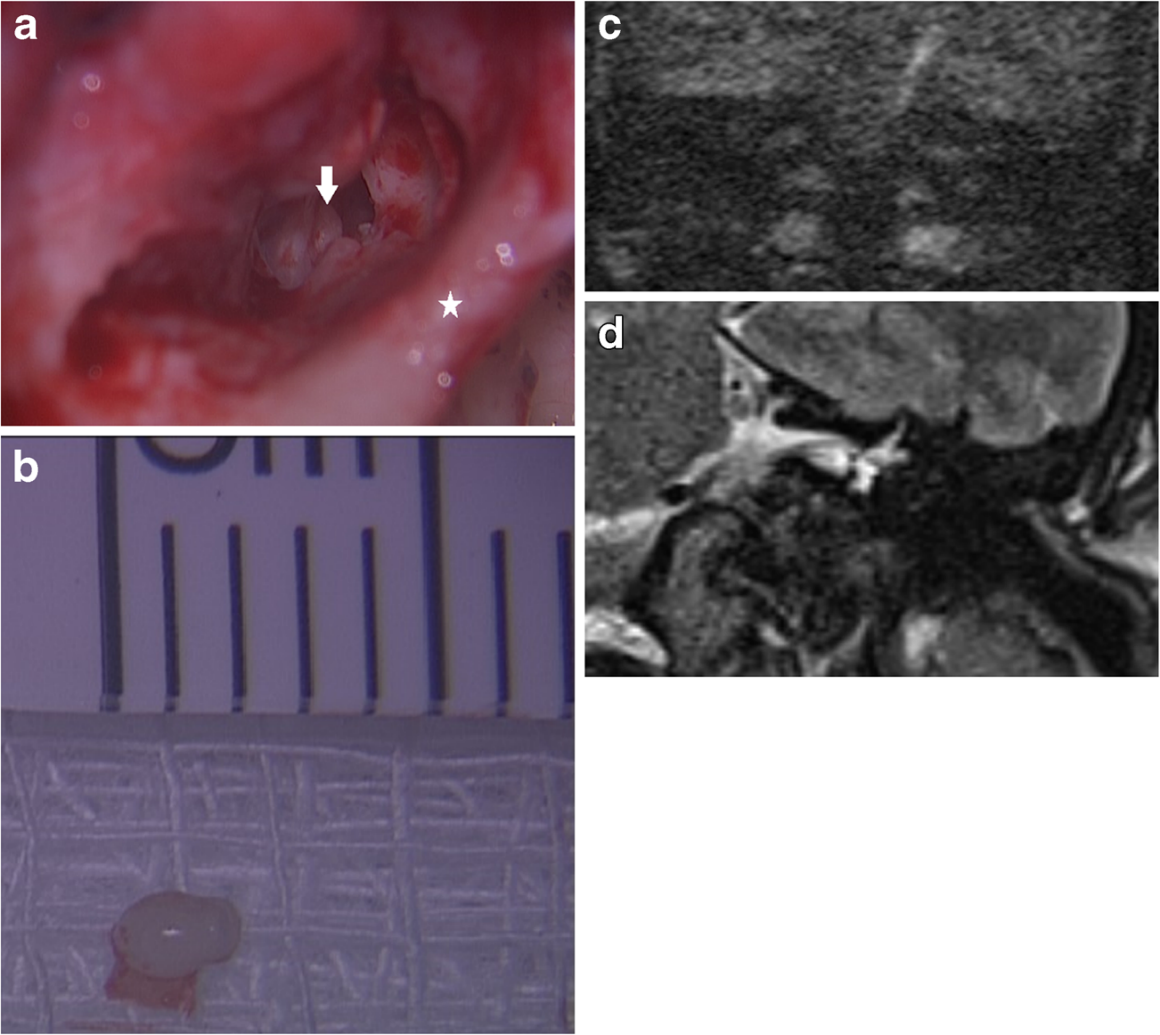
False negative case for cholesteatoma on DWI. a, b A small 2 mm cholesteatoma pearl (*white arrow*) was found on surgery in the hypotympanum *(star denotes posterior canal wall)*, but was not depicted on c the corresponding 2 mm thick coronal b1000 image or d T2 weighted image
